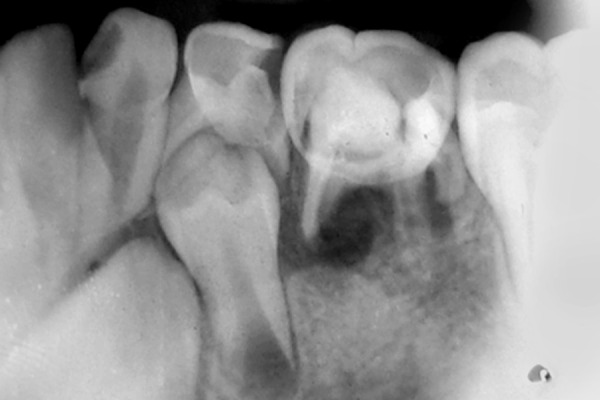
IOPA X-ray showing 85 and missing 45
